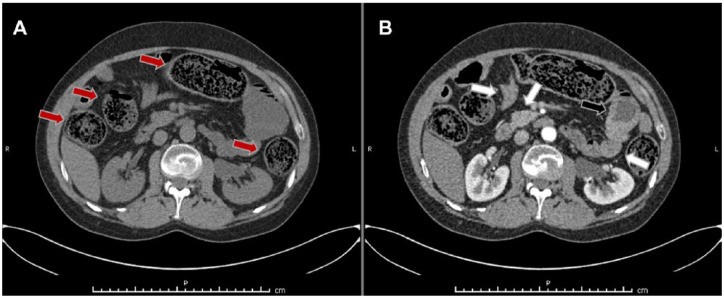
Abdomen computed tomography (CT) scan during the fifth episode of acute intestinal pseudo-obstruction. (a) and (b) CT scan images acquired before and after contrast administration. Abdominal computed tomography (CT) scan reveals diffuse distension of intestinal loops (red arrows) that dislocate and compress the liver. Diffuse hyperenhancement after contrast administration can be observed in the small intestine (white arrows) along with thickening (max 8–9 mm) of the intestinal wall (black arrow).
